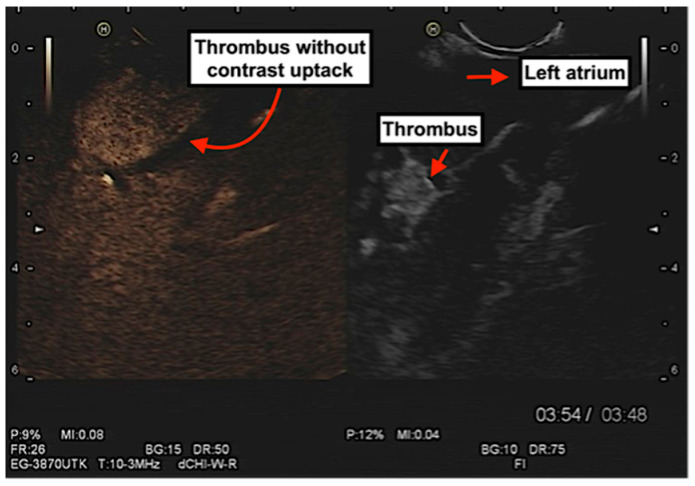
Contrast endosonographic ultrasound demonstrated the size and morphology of thrombus in left atrial appendage and its hypo-enhanced characteristic in the same patient.
